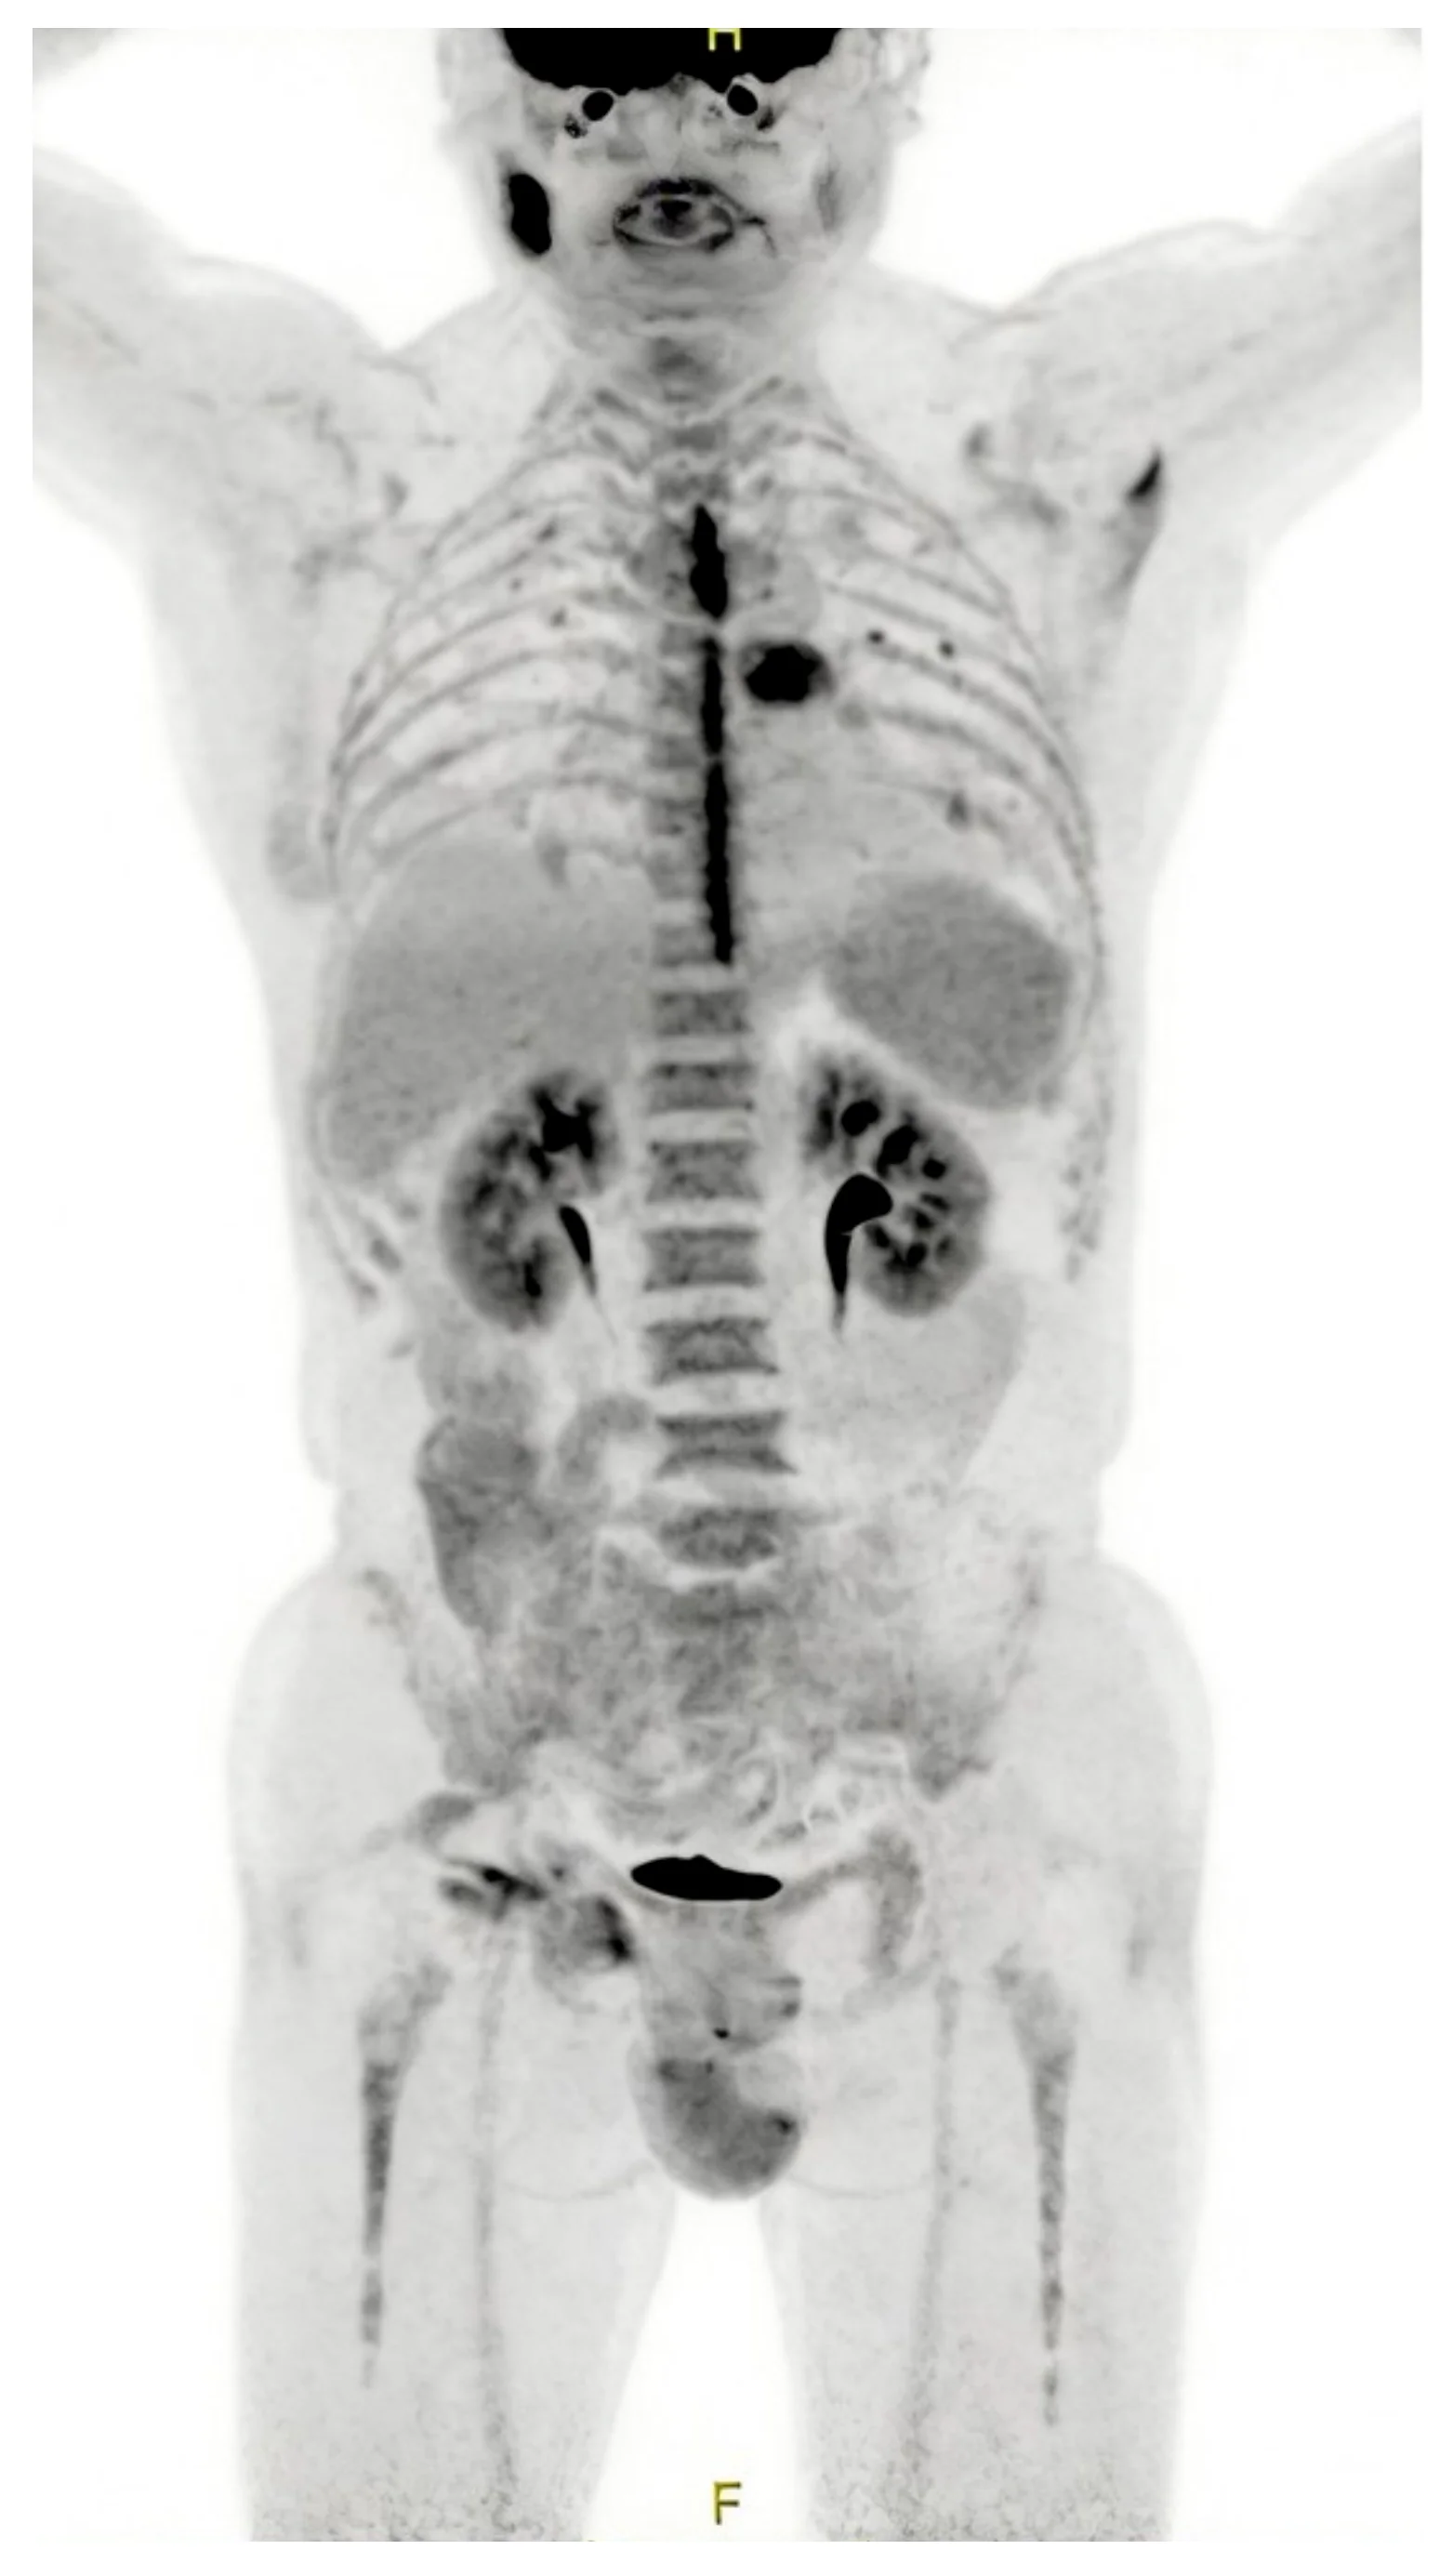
PET/CT. A high-density shadow in the proximal pulmonary artery trunk (3.2 cm × 3.4 cm), SUVmax 9.1, and multiple solid nodular shadows in both lungs, SUVmax 2.1–3.7, were considered infectious lesions. The letter F denotes fluorine-18 in 18F-FDG.
